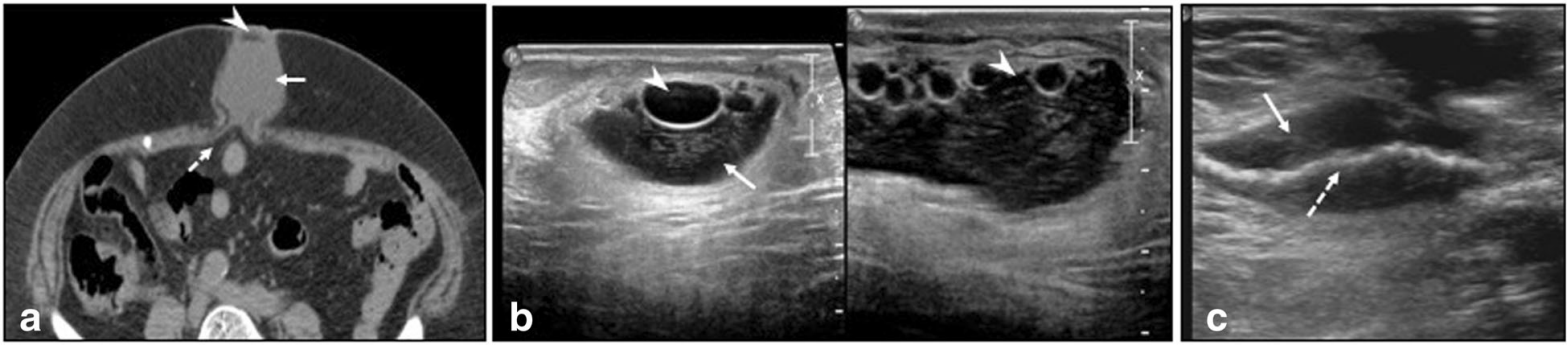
Varying appearances of seroma post hernia repair. a Plain CT in axial section shows seroma (solid arrow) with fat levels (arrowhead). Dashed arrow is the mesh. b USG shows floating fat locules (arrowheads) secondary to tissue dissection within the seroma (arrow). c USG shows seroma (solid arrow) around the mesh (dashed arrow) in an early postoperative hernia repair
